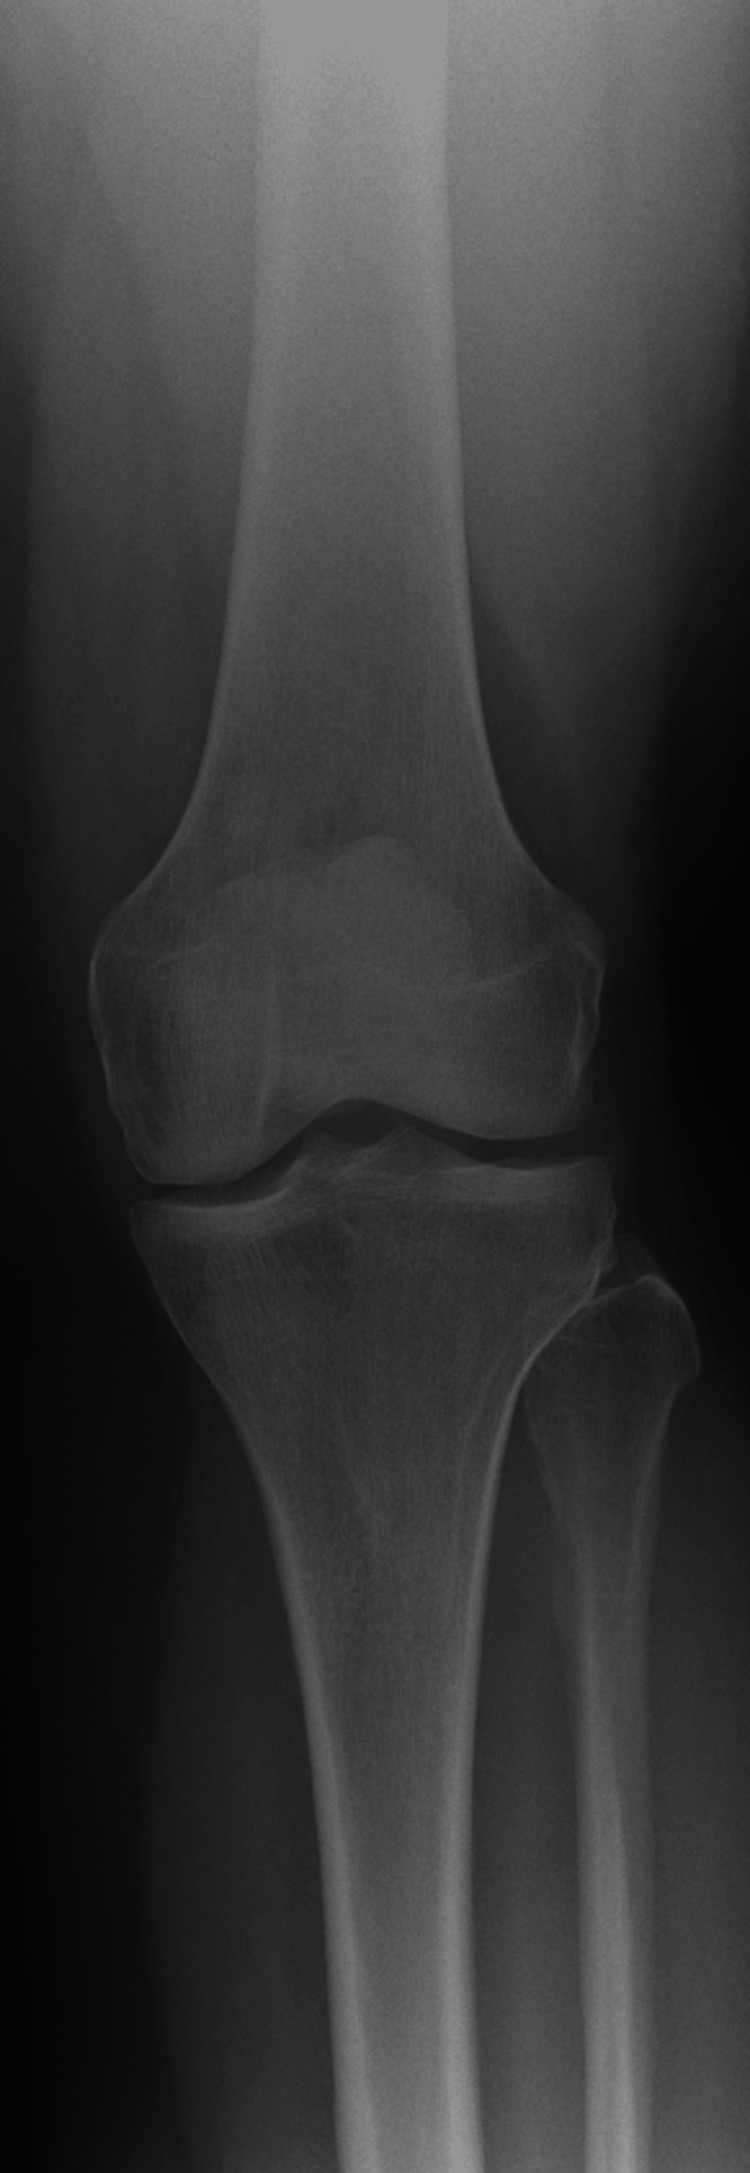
Representative radiograph of Kellgren-Lawrence grade 2 knee osteoarthritis Anteroposterior weight-bearing radiograph showing some osteophyte formation with joint-space narrowing and early subchondral sclerosis. These features meet the criteria for Kellgren-Lawrence grade 2 mild osteoarthritis.
